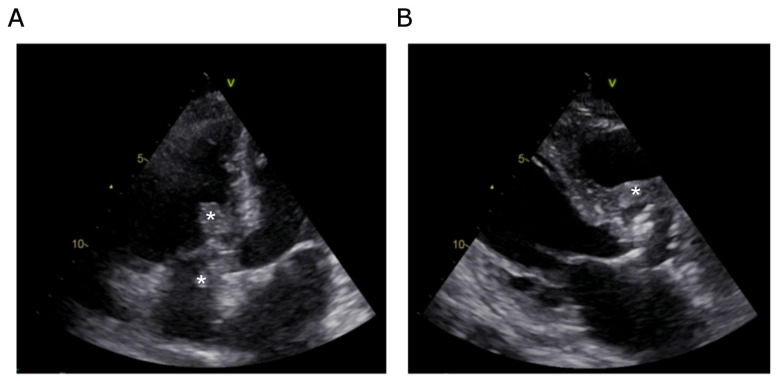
Two-dimensional transthoracic echocardiography images. (A) Apical view demonstrating two masses attached to the tricuspid valve, one on the atrial side and one on the ventricular side (asterisks). (B) Parasternal long-axis view showing thickening of the anterior aortic annulus, suggestive of abscess formation (asterisk). The aortic valve appears severely calcified, and the presence of a vegetation at this level cannot be excluded.
